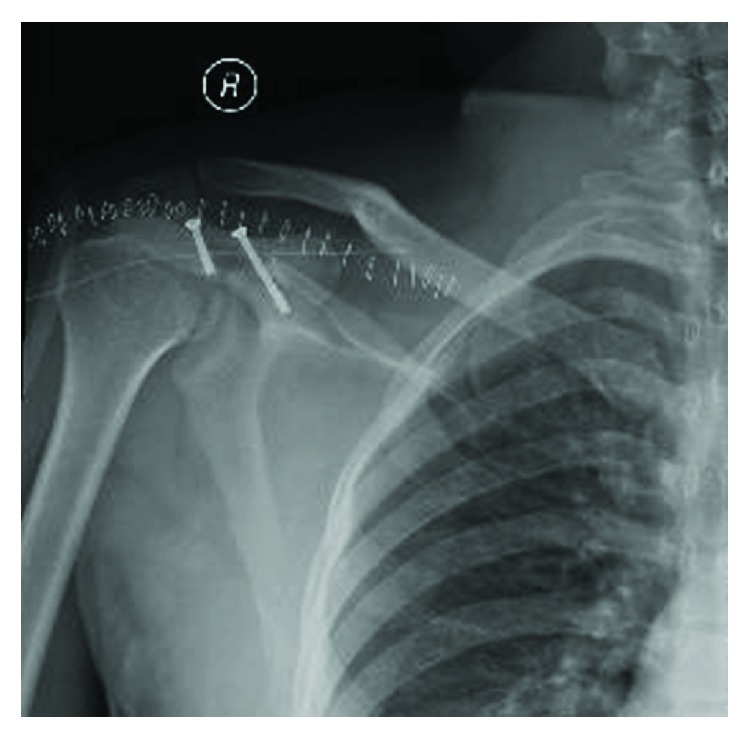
Anteroposterior X-ray of the right shoulder.
